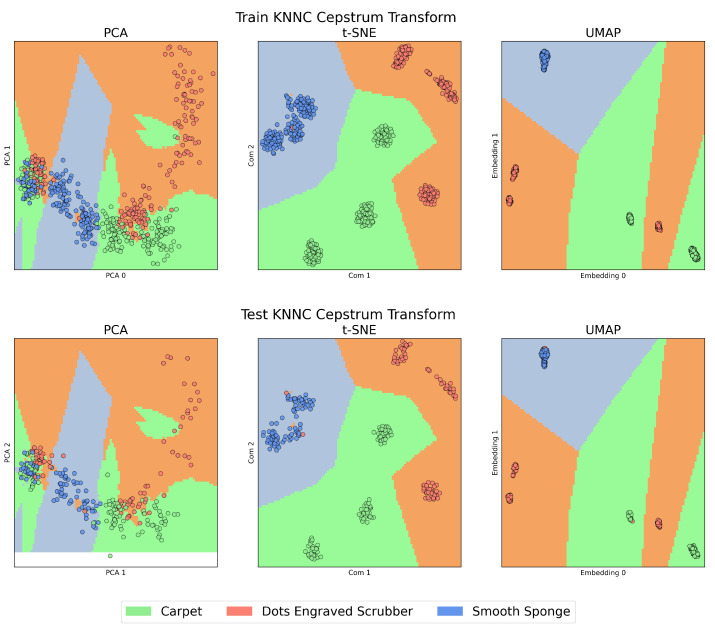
Clustering performance with k-NNC. Comparison of training and testing using k-NNC on PCA, t-SNE, and UMAP for CT signals.
